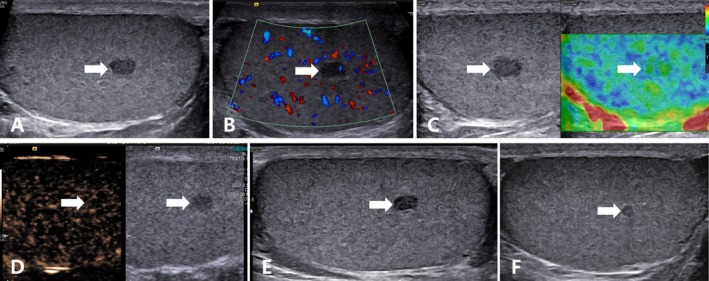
A 39‐year‐old male who was known to have pulmonary sarcoidosis attended the urology clinic complaining of intermittent right‐sided dull testicular ache. A, Greyscale ultrasound of the right testis showed hypoechoic lesions (the largest measuring 4 mm, arrow). B, Color Doppler ultrasound (CDUS) image demonstrated avascularity within the lesions (arrow). C, Strain elastography (SE) showed a lesion with moderate stiffness (arrows). D, Contrast‐enhanced ultrasound (CEUS) revealed iso‐enhancement (arrows). E, An ultrasound follow‐up showed stable appearances at 3 months (arrow). F, An ultrasound follow‐up showed a decrease in number and size (the largest size: 2 mm, arrow) at 2 years.
